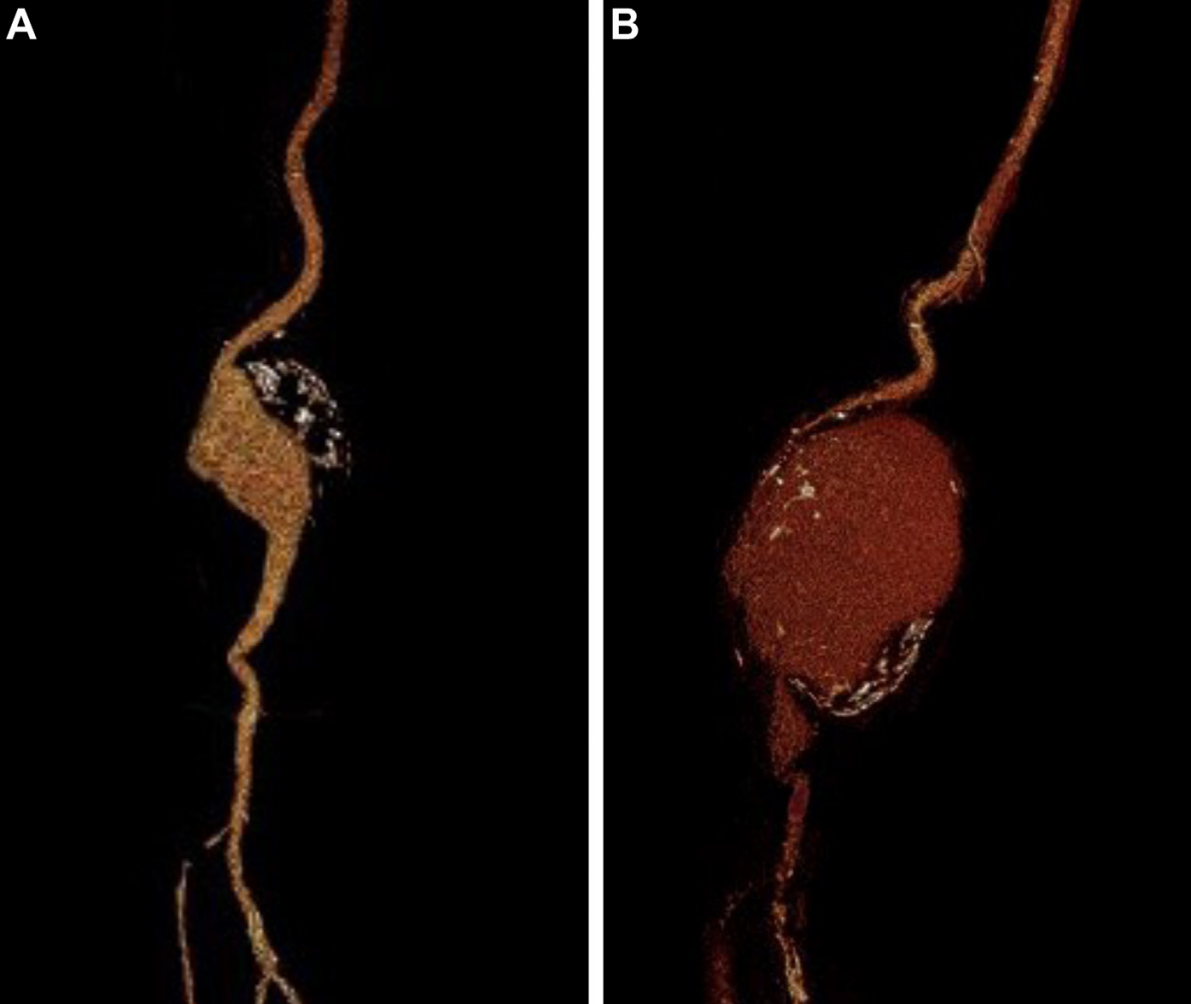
Three-dimensional reconstructions of the right popliteal artery aneurysm (PAA) demonstrating rapid expansion from 46 mm (A) to 81 mm (B) within a 2-week period. The aneurysm diameter was measured on axial images perpendicular to the flow line to the largest diameter of the vessel with three-dimensional reconstructed computed tomography images.
